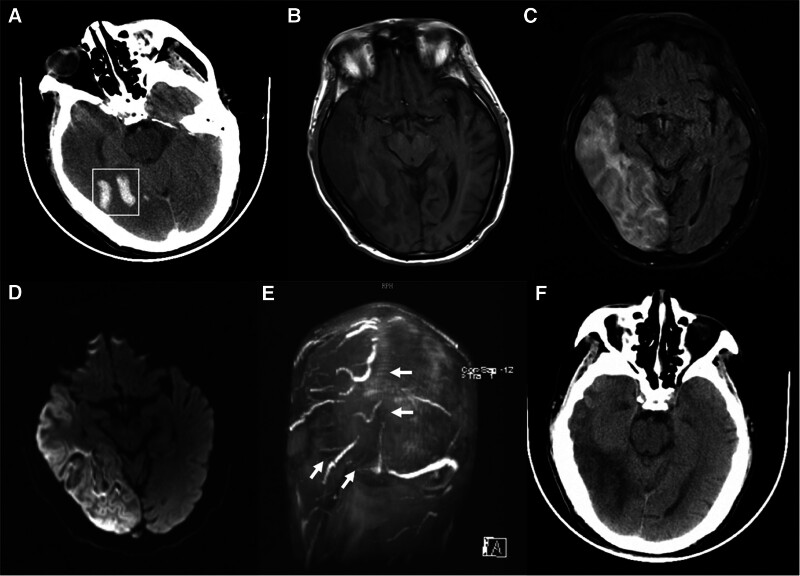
Case 1. (A and B) Non-contrast brain computed tomography (CT) scan and T1 hyperintensity of magnetic resonance imaging (MRI) suggest symmetrical crescent-shaped intracranial hemorrhage in the right temporal and occipital lobes (White box). (C) Fluid-attenuated inversion recovery (FLAIR) imaging shows juxtacortical edema surrounding the hemorrhage. (D) Diffusion weighted imaging (DWI) shows massive cerebral infarction throughout the right temporal and occipital lobes. (E) Magnetic resonance venography (MRV) imaging shows cerebral venous sinus thrombosis of the superior sagittal sinus, right transverse sinus, and sigmoid sinus (arrows). (F) CT examination obtained at 2-month follow-up.
